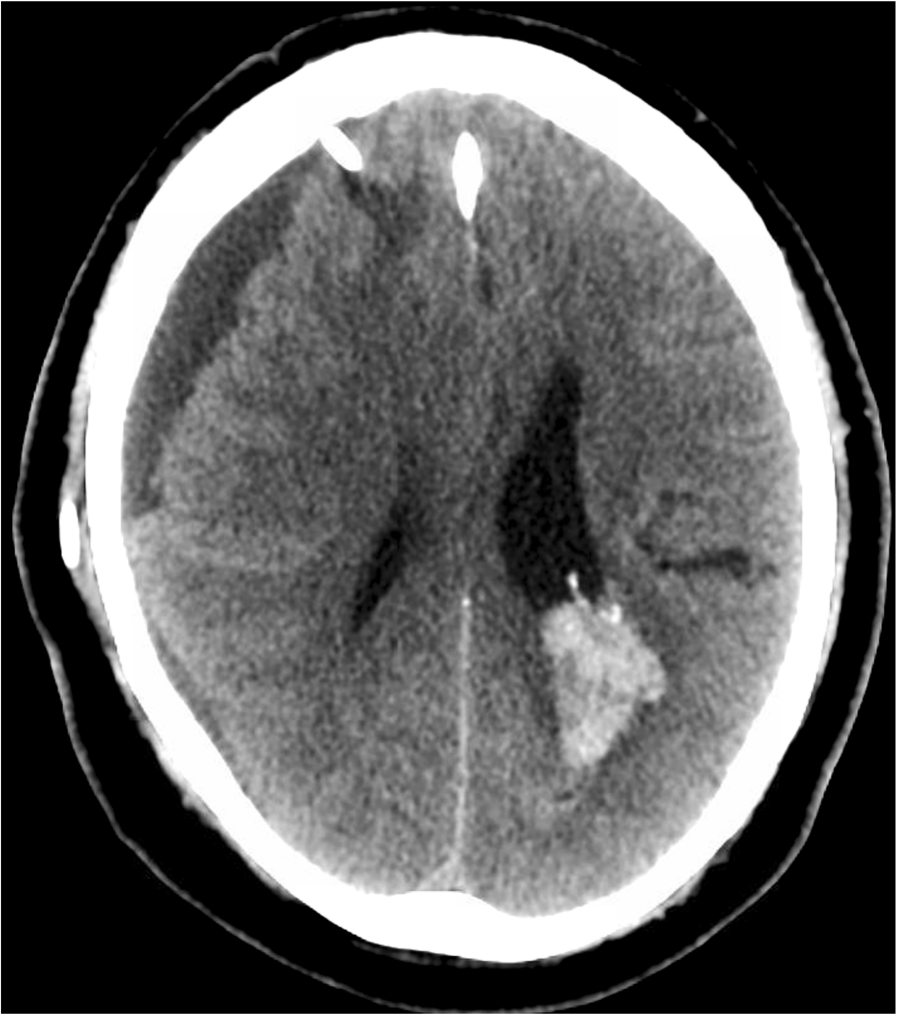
CT head post op day 9, showing interval expansion of hemorrhage and subdural hygroma
